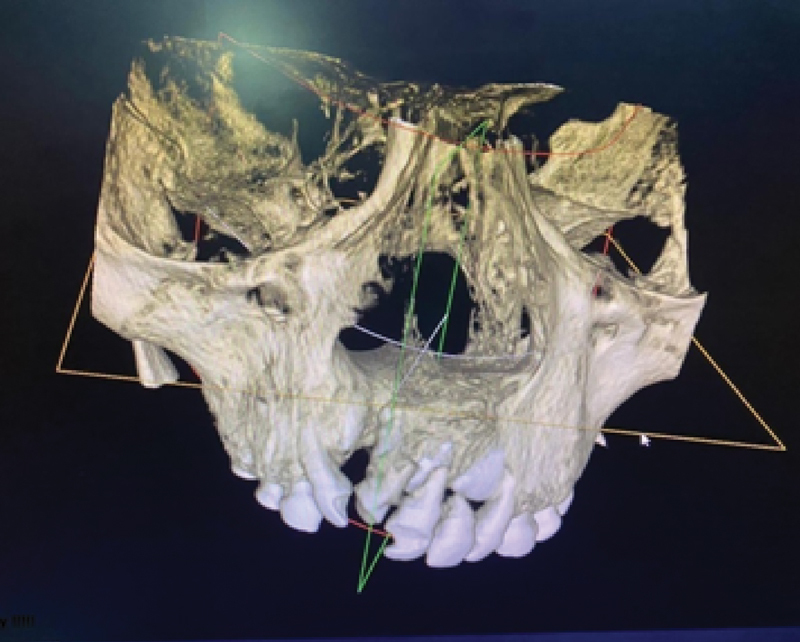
CBCT radiographic assessment of the clefted alveolar bone. CBCT, cone beam computerized tomography.
